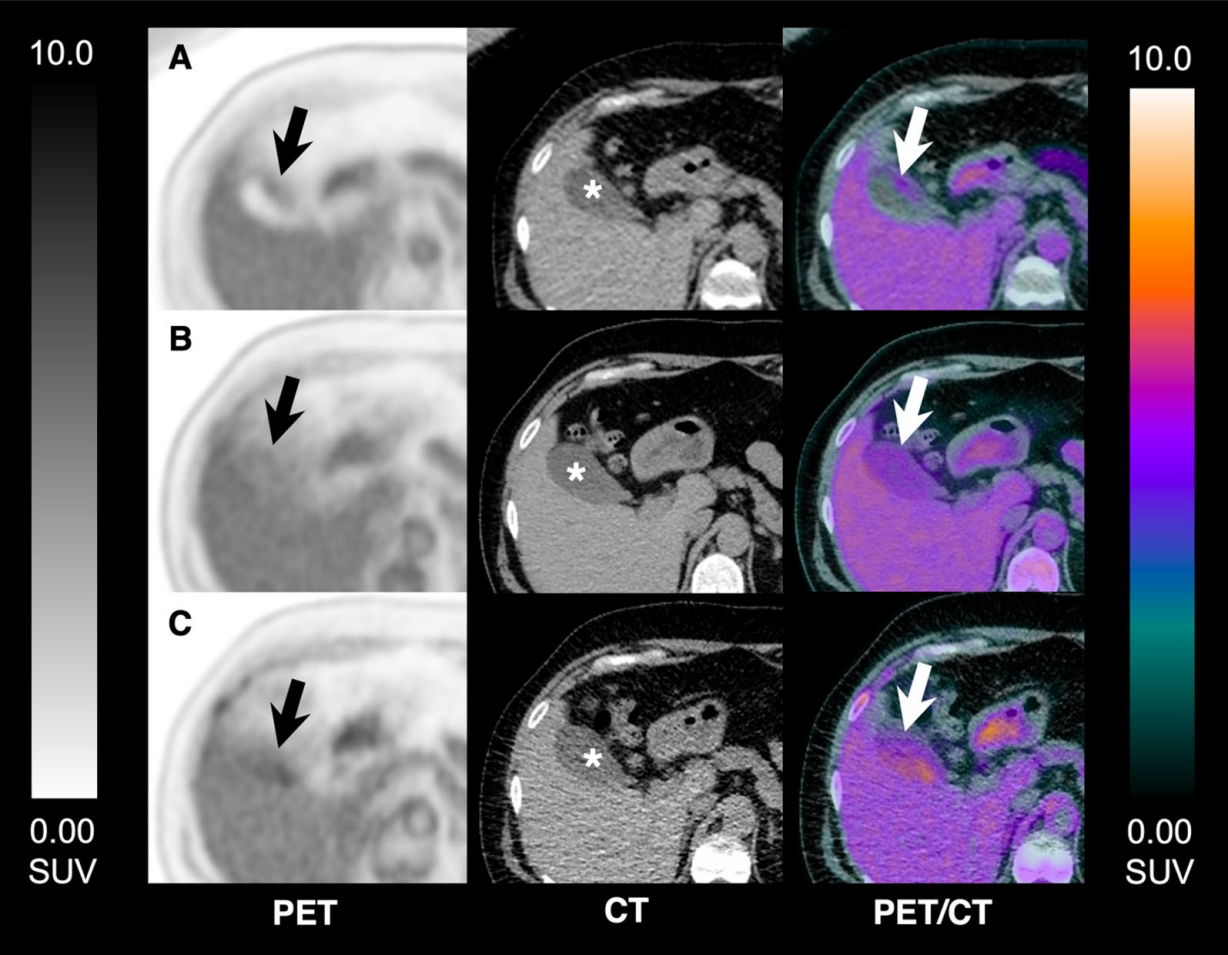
Changes in the gallbladder ^18^F-FDG uptake pattern and intensity on serial total-body PET/CT acquisitions. Axial PET, CT and fused images acquired after i.v. injection of 389 MBq in a 53 y/o healthy participant. Images at 40-min timepoint show wall activity, equal to the liver background; images at 90-min timepoint show luminal uptake, equal to the liver uptake; and images acquired at 180-min post-injection show increased luminal uptake, higher than that of the liver background. Arrows: gallbladder; Asterisks: gallbladder lumen; A PET, CT and fused PET/CT images at 40-min timepoint; B PET, CT and fused PET/CT images at 90-min timepoint; C PET, CT and fused PET/CT images at 180-min timepoint
